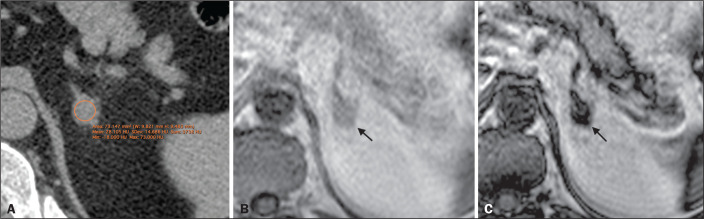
An LPA identified on MRI but not on CT. A: Unenhanced axial CT scan
